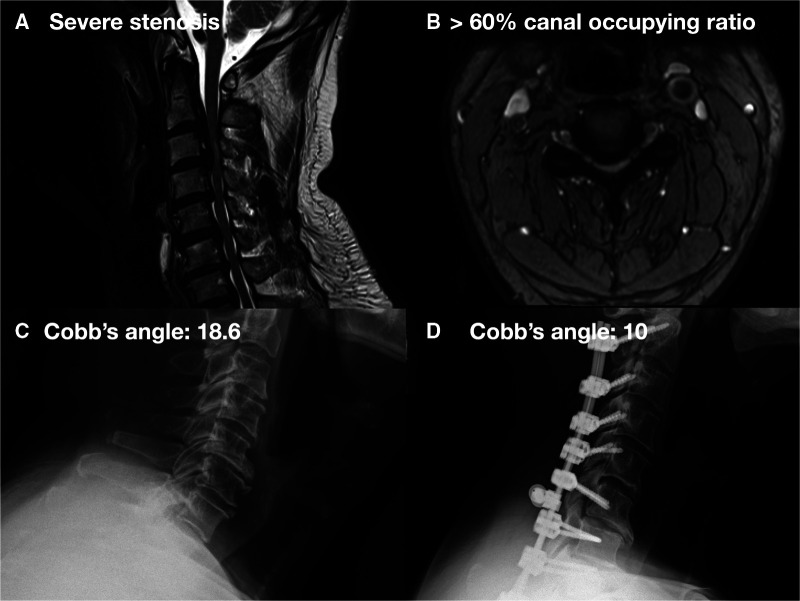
A 51-year-old male with CM due to long-segment massive OPLL over C2-7 underwent C2-7 laminectomy and C2–T2 fixation: (A) preoperative cervical MRI showed severe cervical stenosis with bulging disc. (B) Preoperative cervical MRI axial view showed >60% canal occupying ratio. (C) Preoperative cervical x-ray showed cervical lordosis about 18.6°. (D) Postoperative cervical x-ray showed cervical lordosis about 10°.
